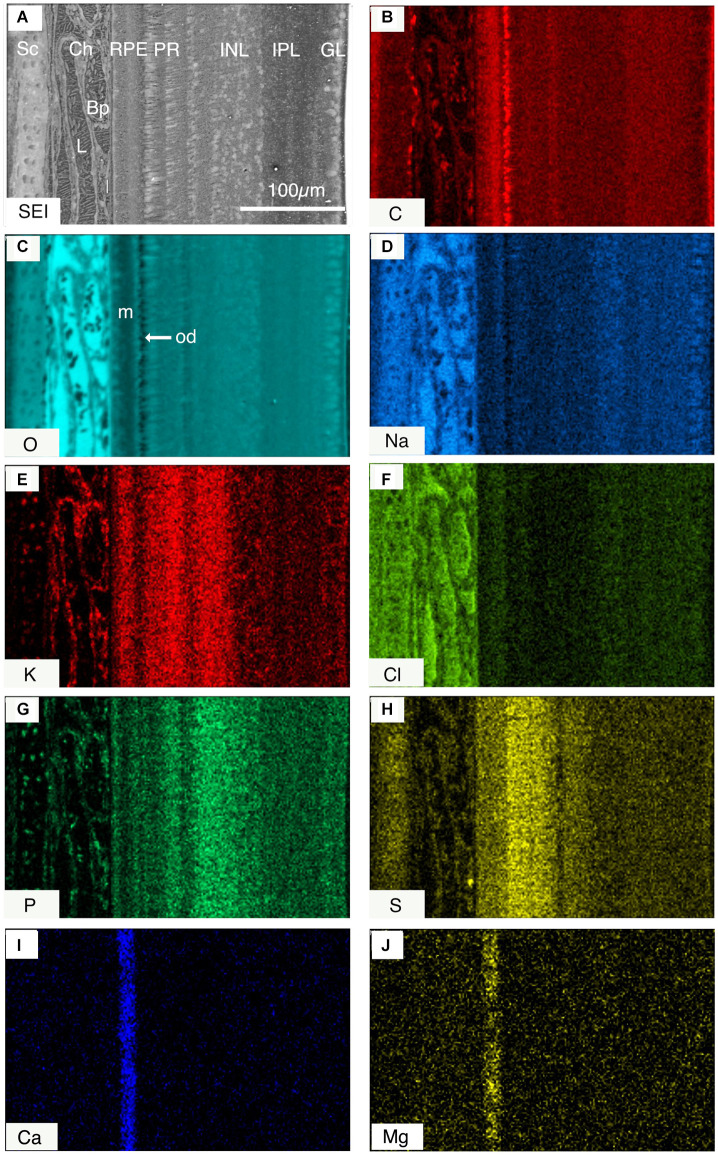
(A) Secondary electron image (SEI) across the frozen-hydrated retinal complex. (B–H) Qualitative images of the relative distribution of different elements (B) carbon C; (C) oxygen O, (D) sodium Na; (E) potassium K; (F) chlorine Cl; (G) phosphorous P; (H) sulfur S; (I) calcium Ca; and (J) magnesium Mg across the posterior eye, where each color represents x-ray intensity (counts) for a different element. The brighter parts of the images indicate higher concentrations of elements. Note (I) calcium, and (J) magnesium are predominantly localized in the melanosome-containing region of the RPE. Abbreviations: Sc, sclera; Ch, choroid; RPE, retinal pigment epithelium; PR, photoreceptor layer; INL, innernuclear layer; IPL, inner plexiform layer; GCL, ganglion cell layer; m, melanosome-containing region of RPE; od, oil droplet.
